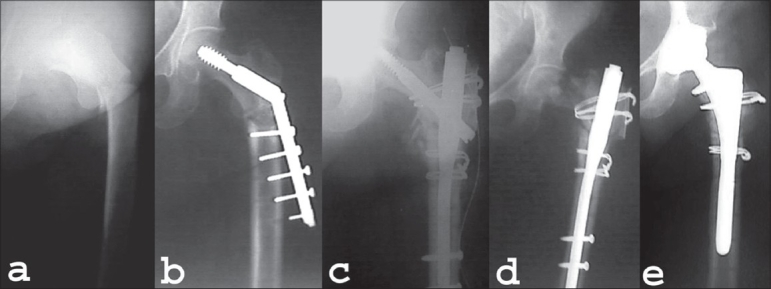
(a) AP radiograph of 58yrs old lady with subtrochanteric fracture of her left hip. (b) Fracture was fixed with DHS which pulled out in 4 months time. (c) DHS was removed and refixation was done with proximal femoral nail. (d) Leg screw of PFN cut through the head and was removed. Fracture went into non-union. (e) PFN removed and uncemented THA performed 20 months after fracture
